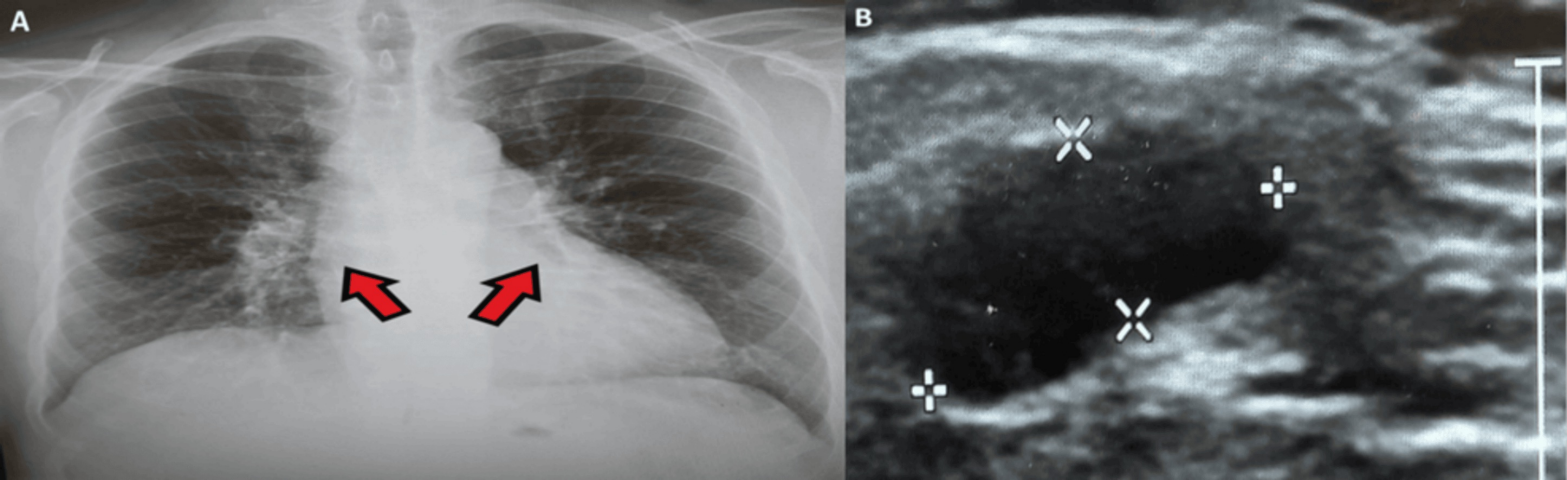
Patient's chest X-ray showing hilar prominence (A) and ultrasound of the neck showing cervical lymph nodes (B)
